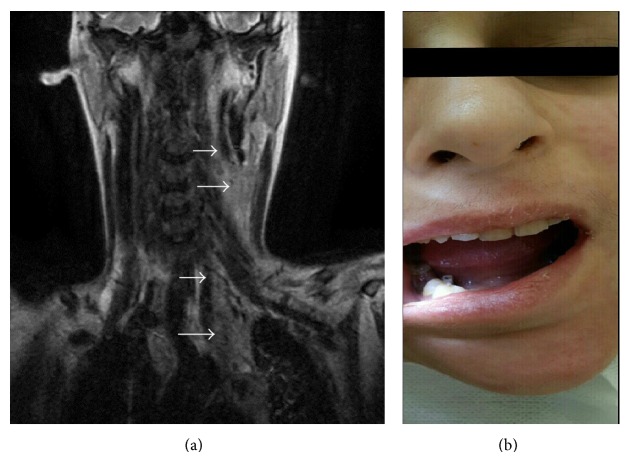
(a) Cervical MRI images (coronal section) that show multiple abscess formation in left carotid space from skull base to the mediastinum and submental lymphadenopathy. (b) The images of the patient after tooth extraction and a Penrose drain application by the consultant dentist.
